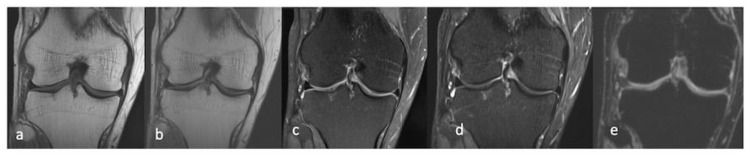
Coronal MRI- T1 (a), PD (b), PDFS (c), STIR (d), T2 med volume (e) of the right knee.
